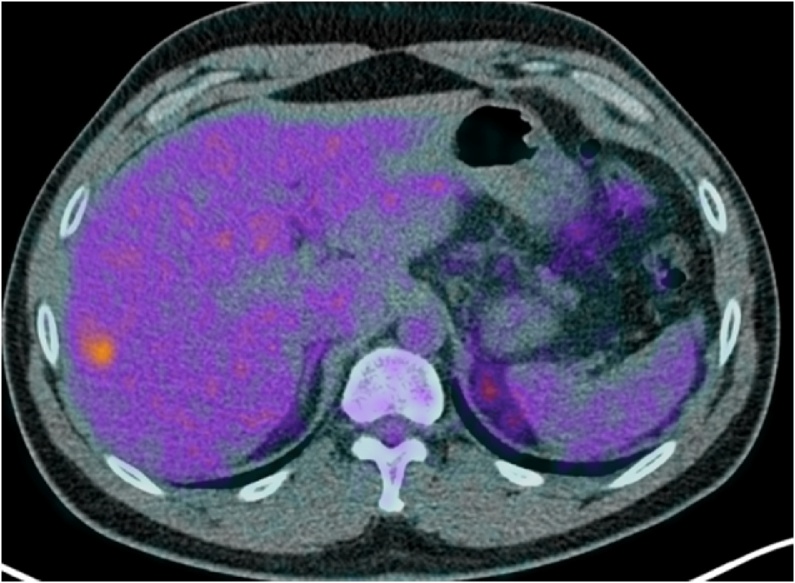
Positron emission tomography–computed tomography after chemotherapy revealed only one hypermetabolic area (SUVmax = 4.2) in liver segment VI.
